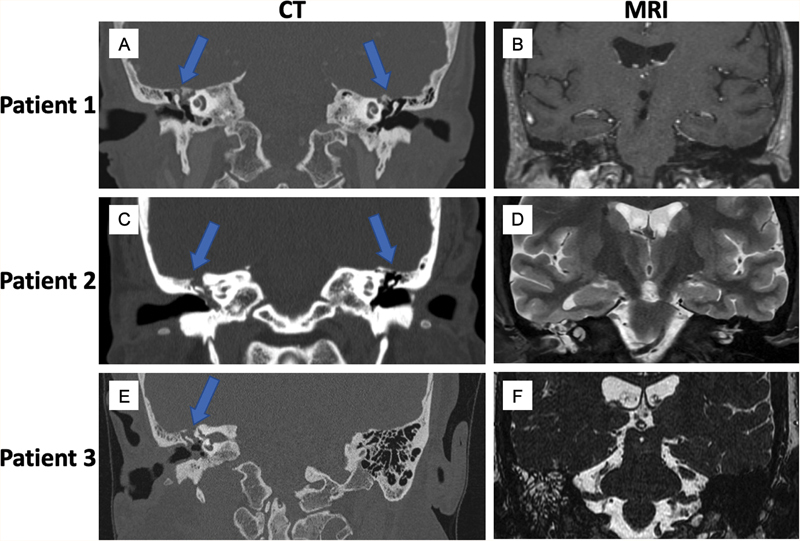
(A, C, E) Preoperative coronal computed tomographies (CTs) demonstrating multiple tegmen defects bilaterally. (B, D, F) Preoperative magnetic resonance imaging (MRI) demonstrating suspected right-sided encephaloceles through tegmen defects.
